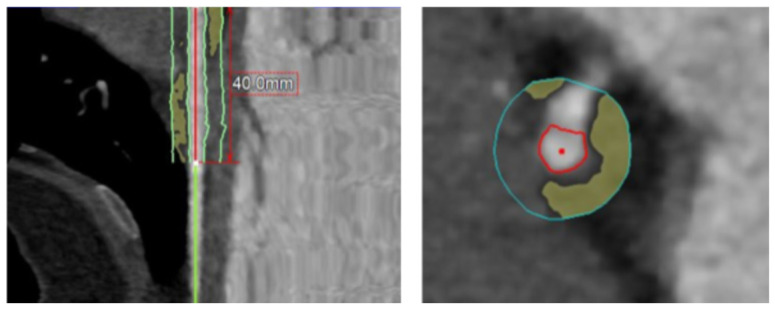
Coronary CT reconstruction of the right coronary artery. Selection of the region of interest analysis showing the distribution of values between −190 and −30 HU (yellow) in long-axis (left) and short-axis views (right). Red lines indicate centerline in long-axis and the perimeter of the coronary artery visualized during the angiographic phase in short-axis.
